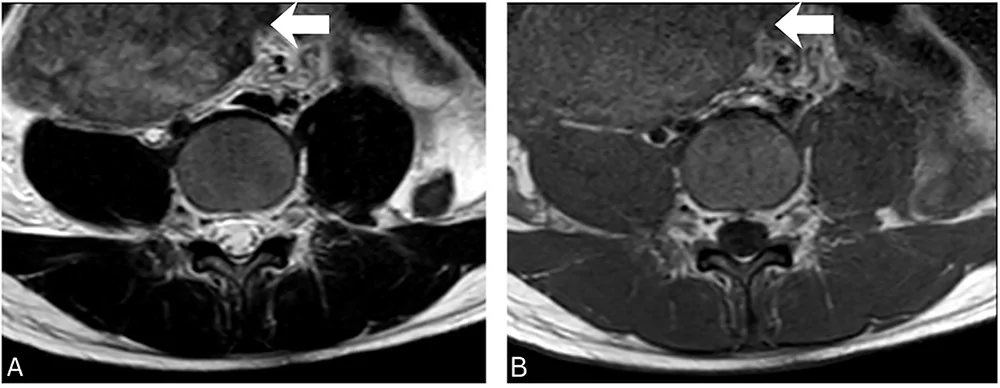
Axial view of a lumbar magnetic resonance image without contrast; (A) T2 weighted image shows heterogeneous intermediate signal intensity (arrow); (B) T1 weighted image (arrow).
